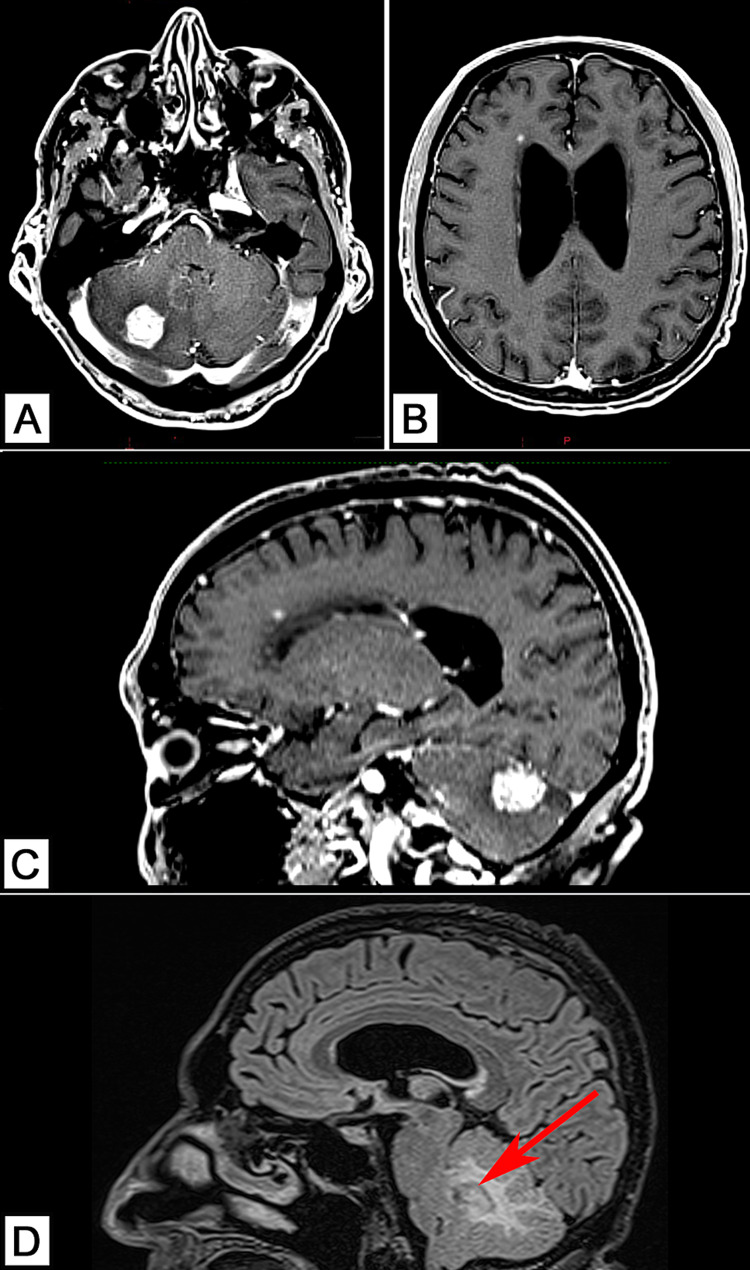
Pre-treatment brain MRI (A) Homogenous contrast-enhancing lesion localized in the right cerebellar hemisphere and causing significant vasogenic edema with mass effect, occluding the fourth ventricle. (B) Small homogenous contrast-enhancing lesion located anterior to the frontal horn of the right lateral ventricle, without any significant signs of edema. (C) Sagittal contrasted MRI showcasing the location of both lesions in the right cerebral and cerebellar hemispheres. (D) Sagittal FLAIR MRI revealing vasogenic edema and occlusion of the fourth ventricle (red arrow).
